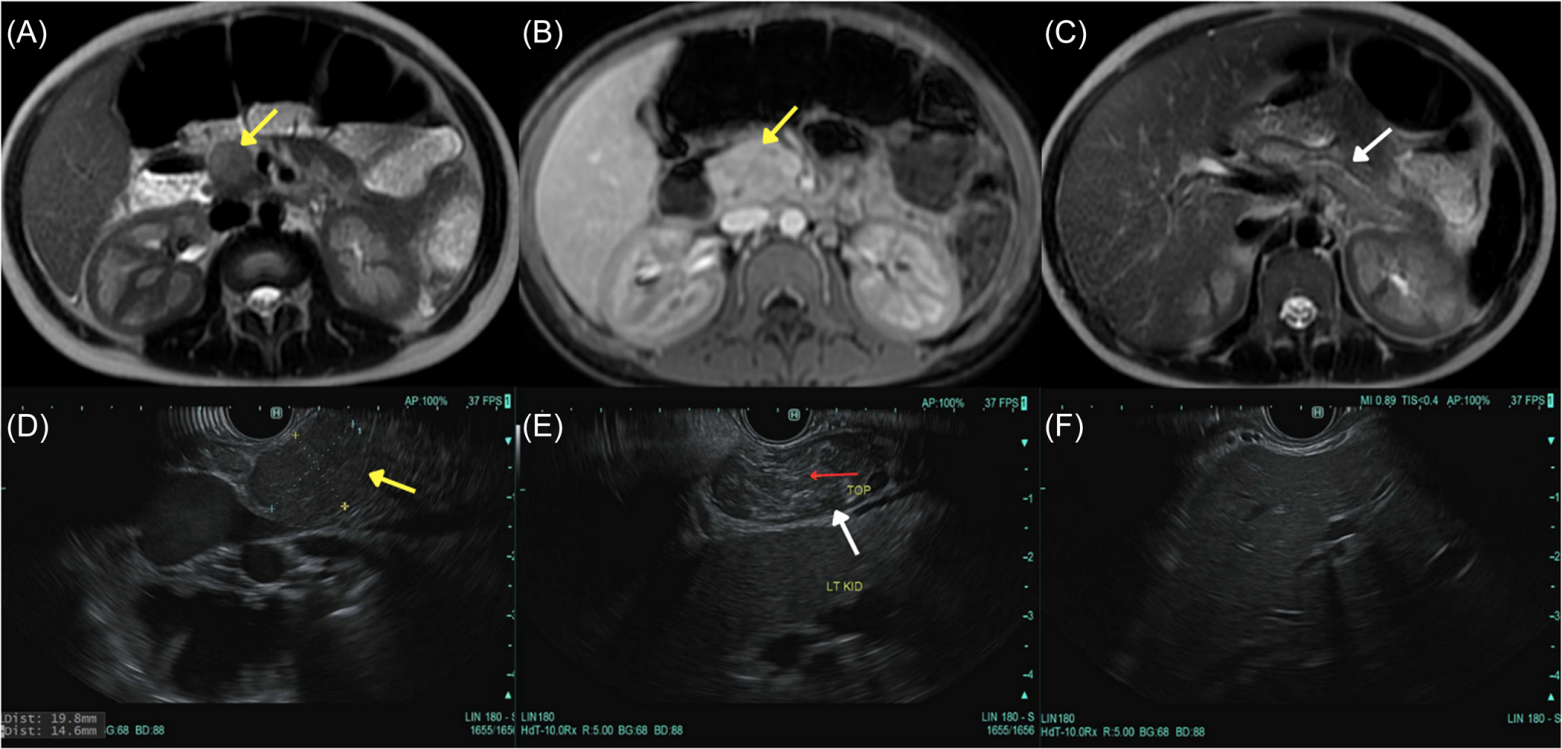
(A) T2 axial MRI section, demonstrating focal mass‐like area (yellow arrow) of high T2 signal in pancreatic head and uncinate process measuring 2.1 ×1.8 cm with no significant associated bile duct or pancreatic ductal distention. There is a thin peripheral hypointense capsule‐like rim on T2 imaging. (B) T1 axial MRI section with gadolinium contrast demonstrating homogenous enhancement similar to normal pancreas throughout the mass like area (yellow arrow). (C) T2 axial MRI section, showing downstream edema throughout the body and tail (white arrow) of the pancreas. (D) EUS image showing an oval, isoechoic and homogenous mass (yellow arrow) at the uncinate of the pancreas, measuring 19 mm in maximal cross‐sectional diameter. (E) EUS image showing the tail (Top‐ white arrow) of the pancreas with normal caliber pancreatic duct (red arrow). The echogenicity is slighly heterogenous described as “salt pepper” with some hyperechoigenic strands which the apperance is considered normal for the patient's age. (F) EUS image showing homogenous parenchyma, with no sign of significant endosonographic abnormality in the left or right lobes of the liver. No focal pathology or masses were identified. This image only shows the left lobe of the liver. There was no sign of significant endosonographic abnormality in the common bile duct. The maximum diameter of the duct was 3 mm. Ducts of normal caliber and with regular contour were identified. No masses were seen. EUS, endoscopic ultrasound; MRI, magnetic resonance imaging.
